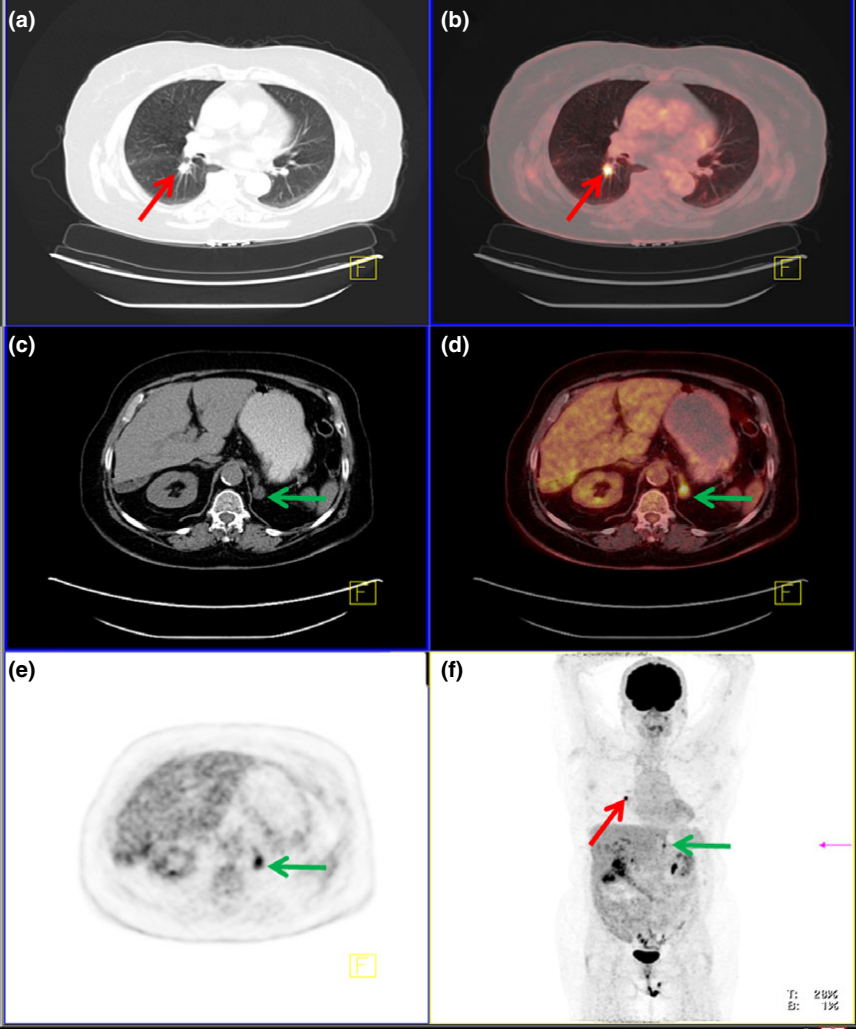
PET/CT scan of a 66-year-old female with a central tumour in the right lung (red arrow), easily visible on CT (a). Tumour was highly FDG-avid indicative of malignancy (SUV_max_ = 16) (b). An enlarged left adrenal gland was visible on CT (green arrow) (c) and similar to the primary tumour highly FDG-avid, corresponding to a metastasis (SUV_max_ = 9) (d,e). Both findings can be seen on the multi-intensity projection (MIP, f) and was confirmed to be adenocarcinoma.
